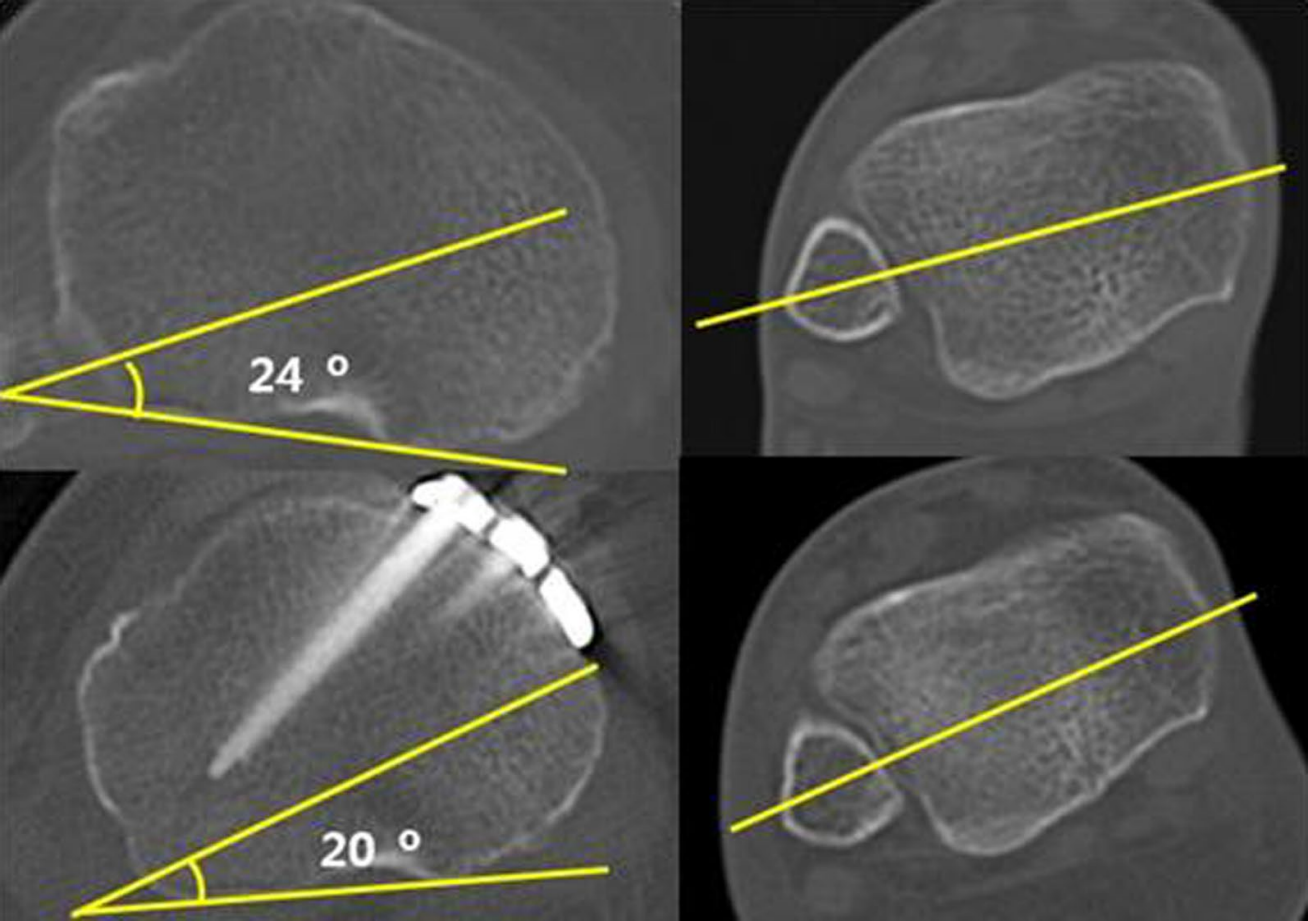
A change in tibial torsion angle (TTA) pre- and postoperation on axial computed tomography was measured. TTA, an angle formed by the axis through the posterior tangent of the widest proximal tibial condyles to the transverse axis that bisects the anteroposterior (AP) diameter of the lower end of the tibia and passes through the anterior half of the lateral malleolus in the axial plane, using computed tomography (CT)
